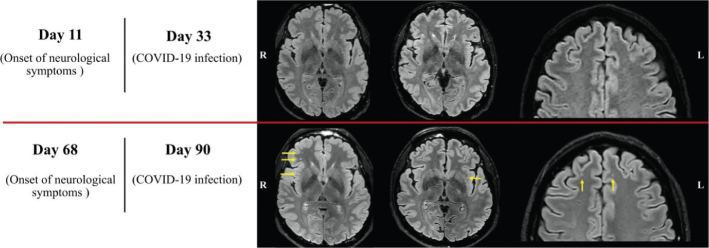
The trend in neuroradiological detection of white matter changes in our patient is summarised. FLAIR images from the second (top row) and the third (bottom row) MRI examinations. Several new subcortical white matter changes were found and are highlighted with yellow arrows. The discrepancy was not considered to be explained by the slight difference in image quality. All other sequences in all three MR examinations showed normal findings.
